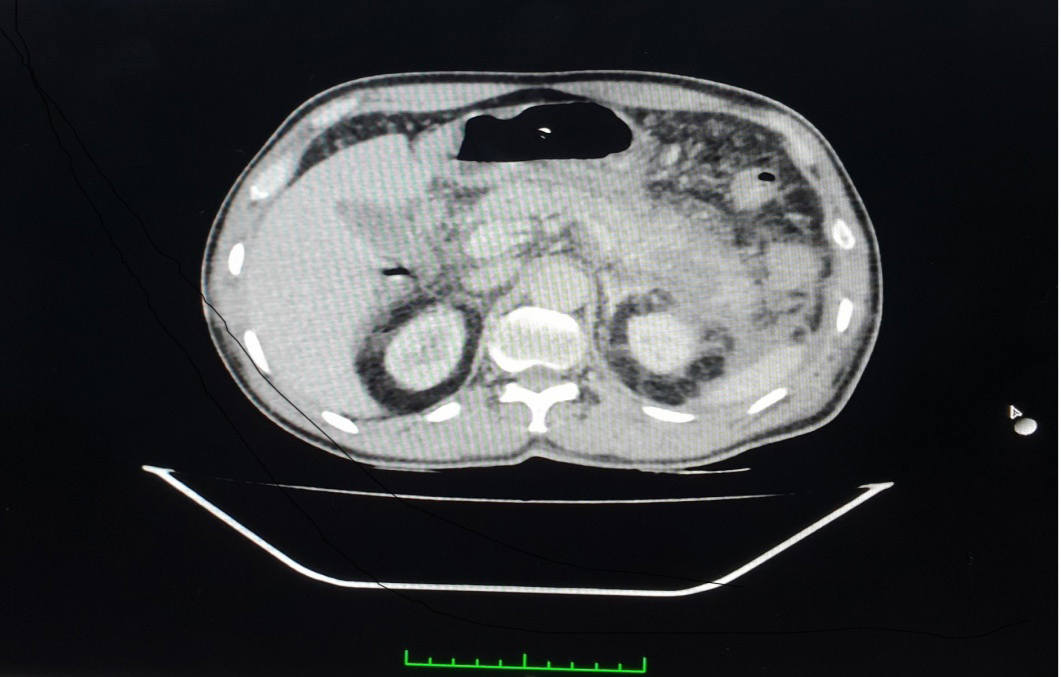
Contrast-enhanced computed tomography of abdomen shows pancreatic necrosis in 30% with swollen neck and tail, peripancreatic inflammation and fluid collection.
